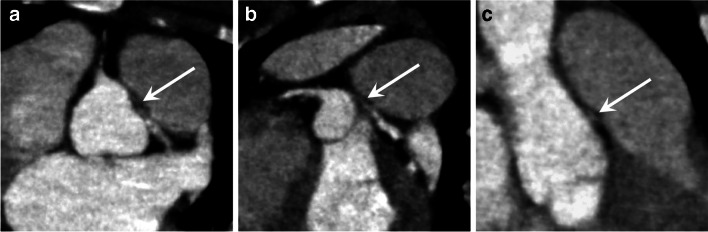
A 12 -year-old boy with an anomalous origin of the left coronary artery from the right aortic sinus who suffered a cardiac arrest while playing basketball. a, b Multiplanar oblique contrast-enhanced cardiac computed tomography (CT) reconstructions show the anomalous origin of the left main coronary artery from the right aortic sinus arising at an acute angulation with an interarterial course. There is a very thin poorly opacified intramural segment (*arrows*) that crosses across the right-left aortic commissure. c Coronal cardiac CT reconstruction shows the interarterial portion in cross section (*arrow*), which is slit-like with loss of pericoronary fat, typical signs of an intramural segment
